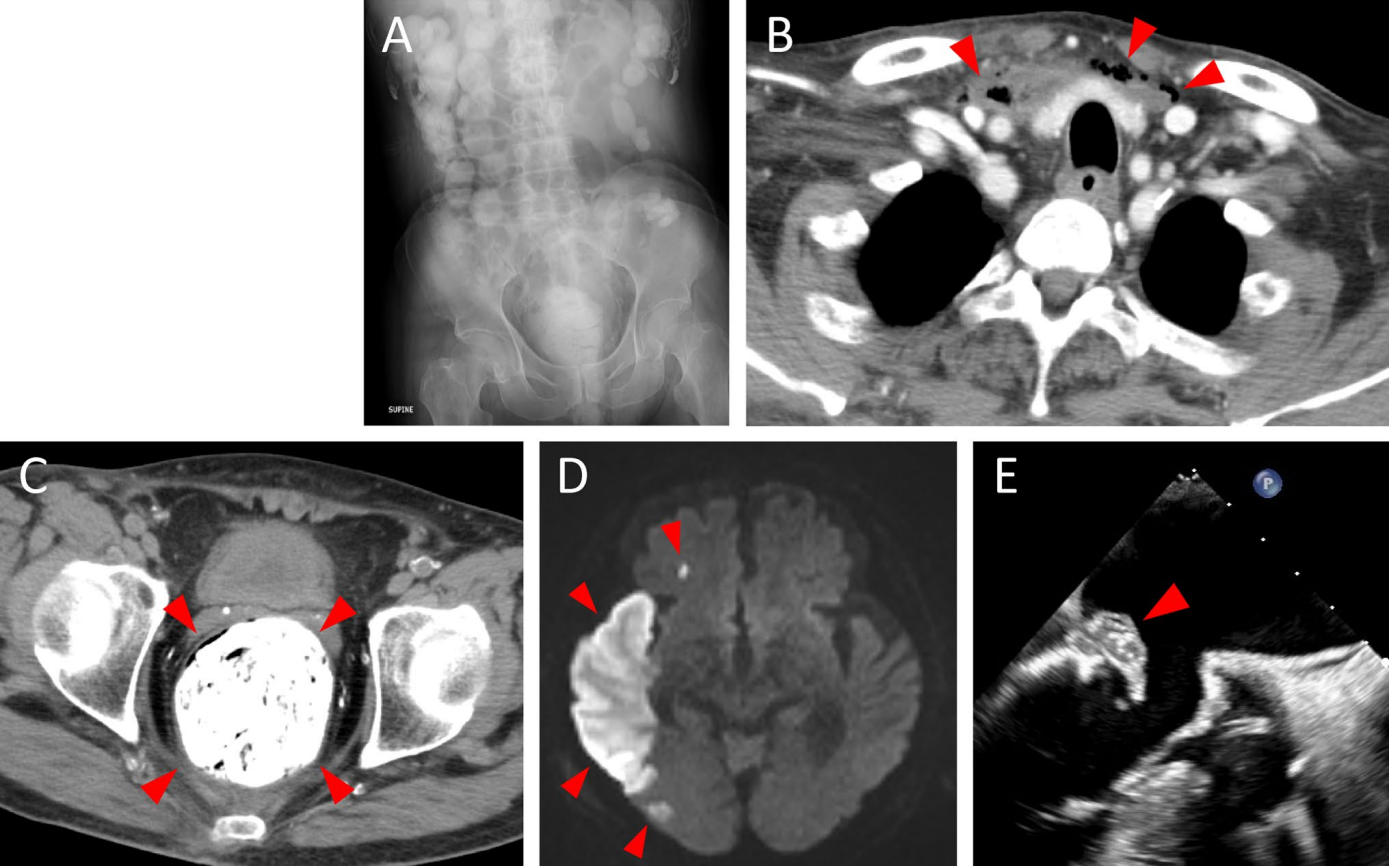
Radiological and ultrasound findings of the patient. A Abdominal radiography showing severe constipation. B Chest computed tomography showing gas production along the anterior mediastinum (arrowheads). C Abdominal computed tomography showing hard stools at the rectal colon (arrowheads). D Head diffusion-weighted magnetic resonance imaging showing multiple cerebral infarctions (arrowheads). E Transesophageal echocardiography showing a 20-mm-sized vegetative structure on the damaged mitral valve (arrowheads)
